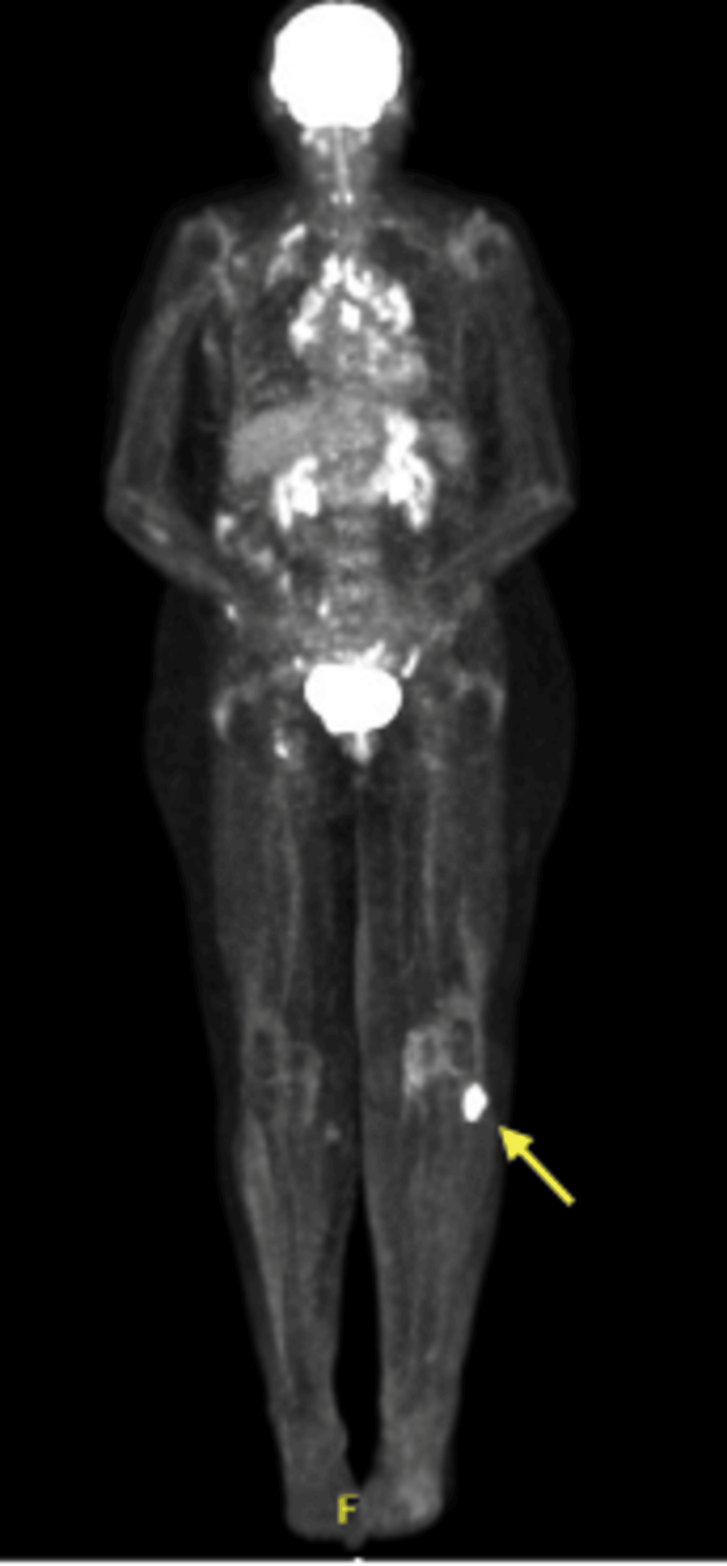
PET-CT scan (coronal view): FDG-avid lytic lesion in the head of the left fibula (arrow)
